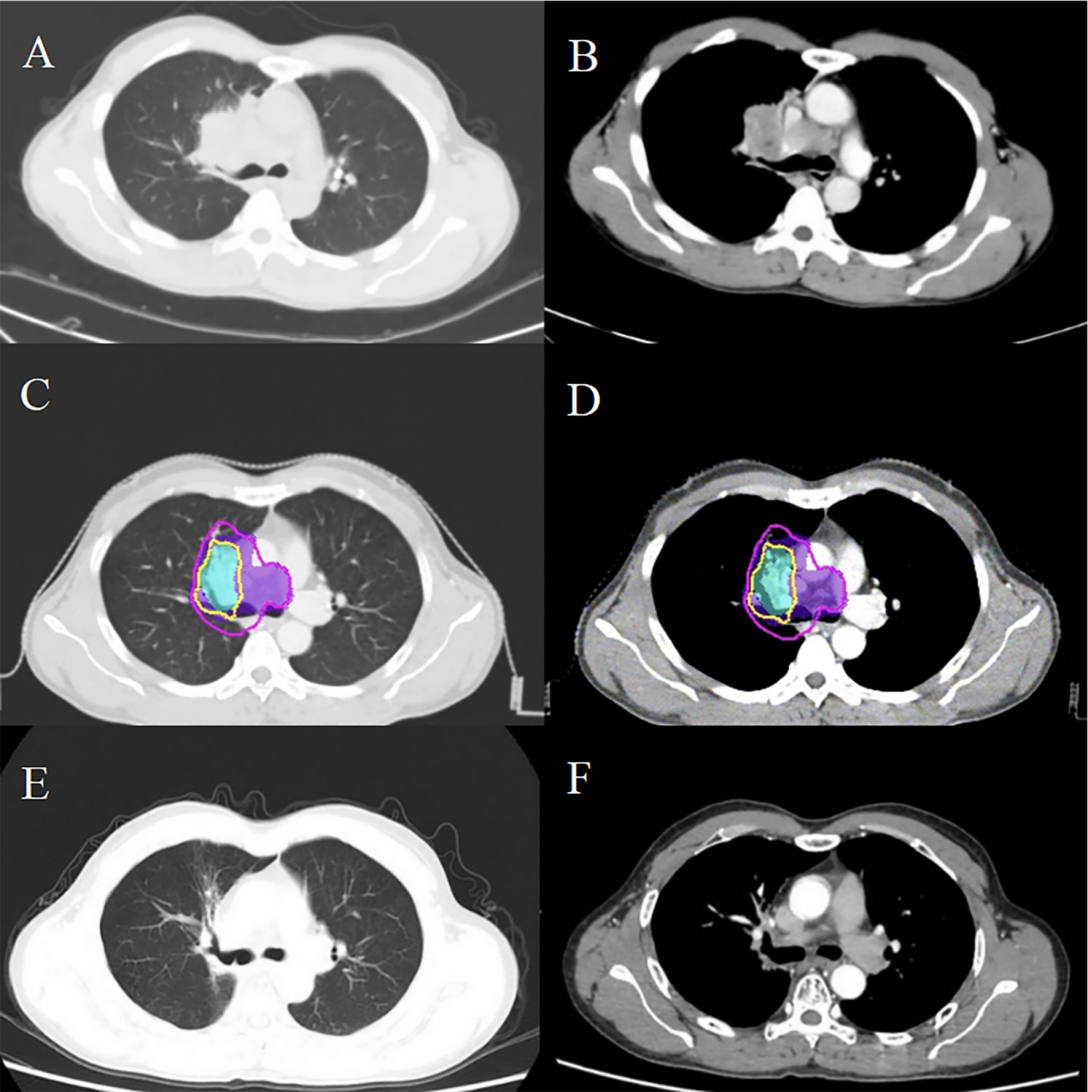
Comparative imaging analysis delineates post-radiotherapy metastatic progression in thoracic regions. Post-treatment surveillance CT identified metastatic lesions in the pulmonary parenchyma (A) and mediastinal nodal stations (B). Dosimetric mapping demonstrates therapeutic coverage with the 60 Gy isodose contour (yellow) delineating the GTV, while the 54 Gy isodose (purple) demarcates the CTV in postoperative imaging series (C, D). Serial follow-up imaging shows sustained locoregional control (E, F), with no radiographic evidence of disease recurrence.
